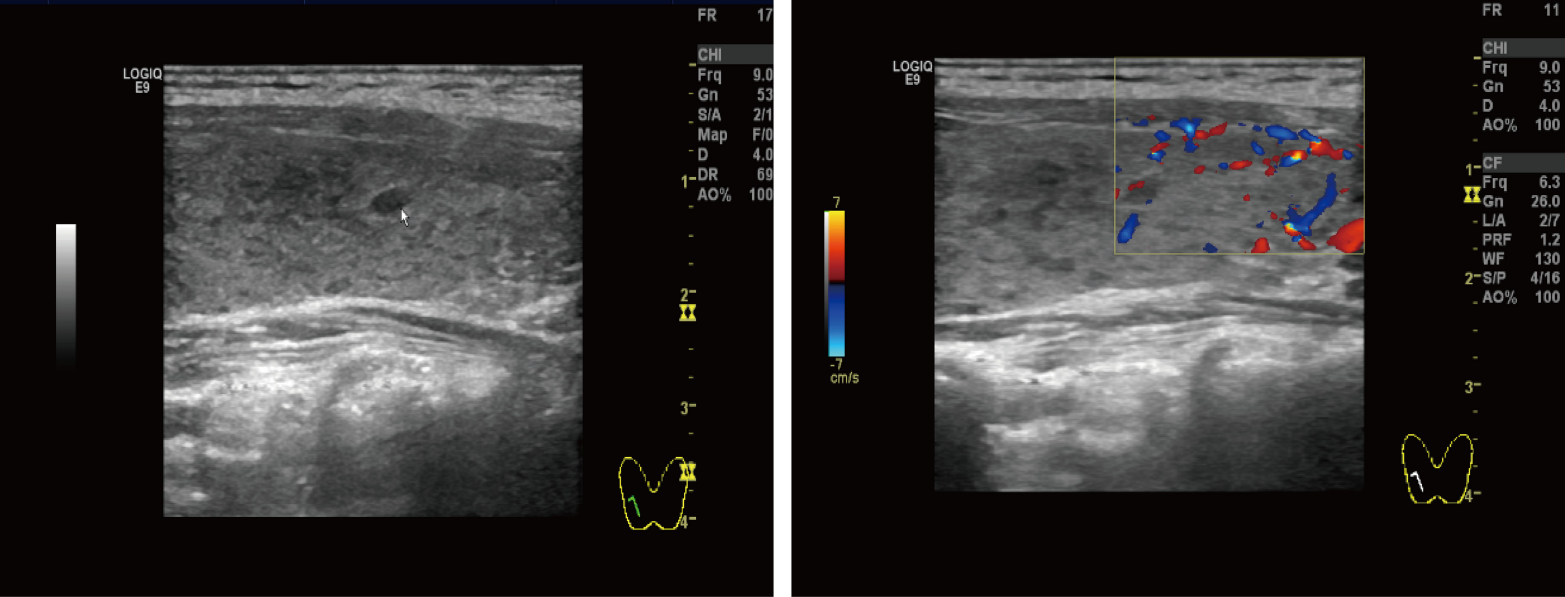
Thyroid ultrasound: Multiple nodules were present in both lobes of the thyroid gland. The larger nodule in the right lobe was located in the middle and lower part, measuring about 4.0 mm × 2.3 mm, with clear borders, regular shape, and substantial hypoechoic nodules. The larger nodule in the left lobe was located in the lower part, with a size of about 3.4 mm × 1.8 mm, with clear borders, regular shape, and substantial hypoechoic nodules.
